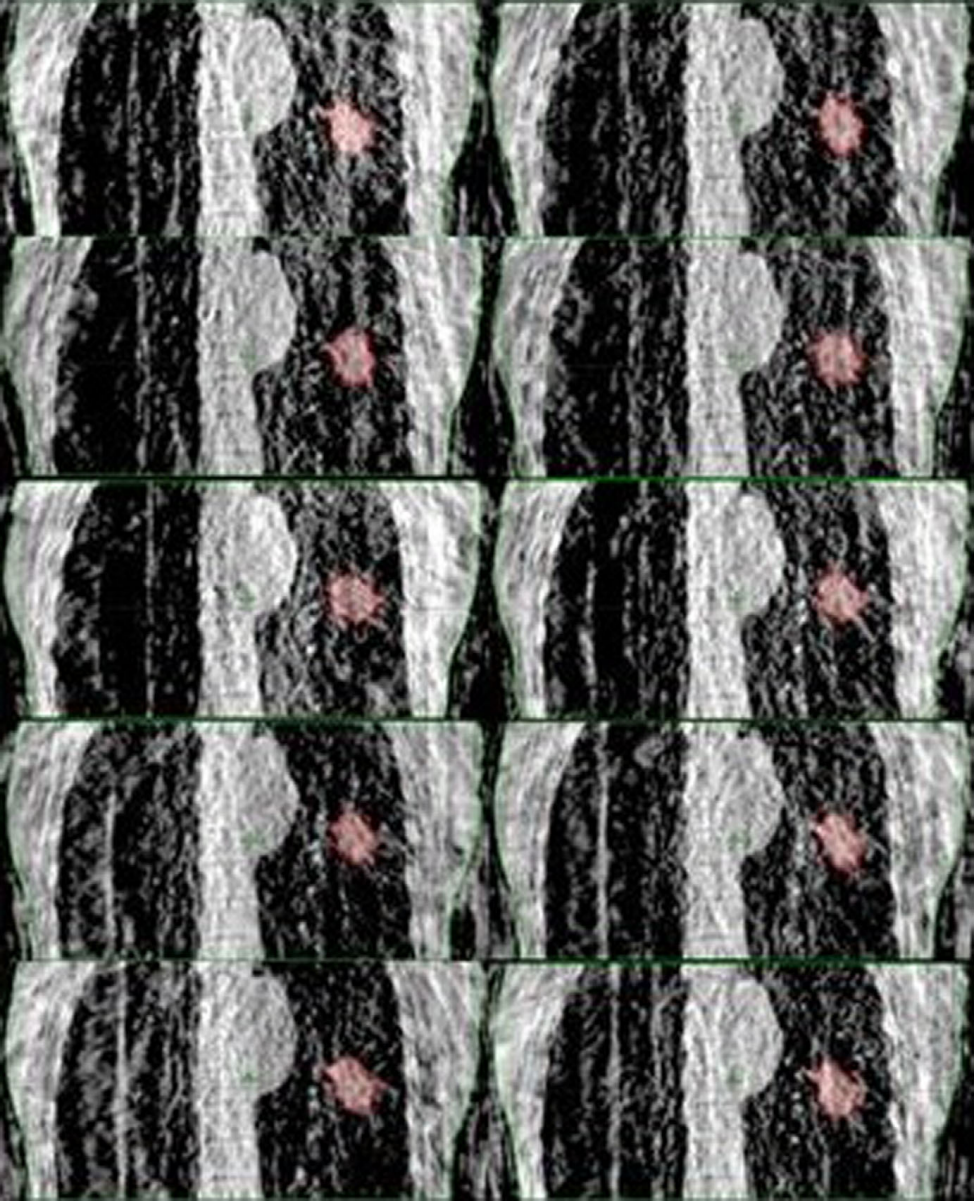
Four dimensional CBCT ITV. Tumor volumes contoured on 10 phases (red). The union of the 10 phases comprised the 4DCBCT ITV.
